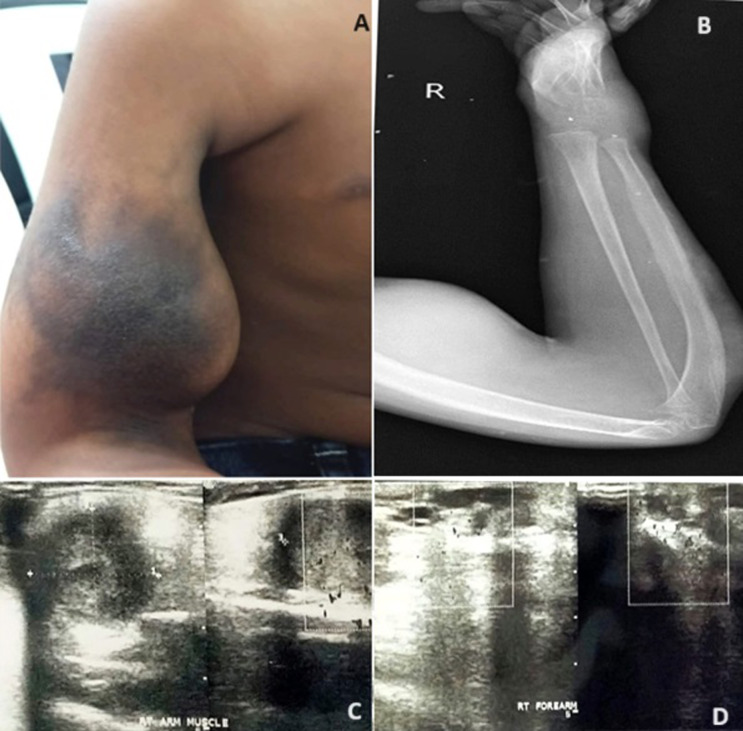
A) vascular malformation on right elbow blackish patch; B) X-ray of the upper forearm shows soft tissue swelling with ill-defined radio densities at the soft tissue plane; C) multiple duct-like structure seen in the right forearm swelling below the posterior aspect elbow; D) large well defined, solid, hypoechoic lesion seen in the intramuscular plane of biceps muscle of right arm (size: 2.7 X 2.3 X 2.3 cm)
